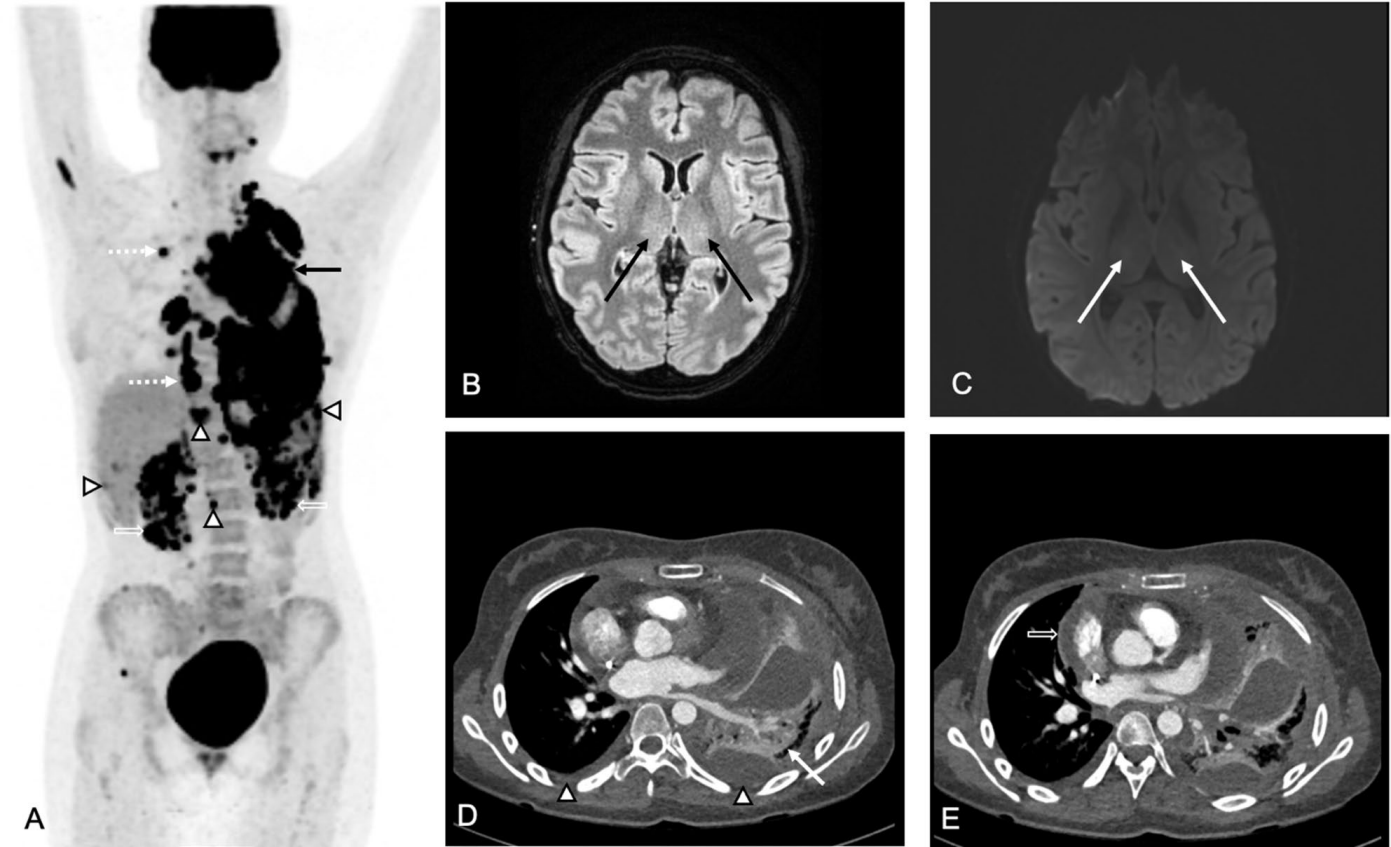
19-year-old female with diffuse large B-cell lymphoma and high baseline metabolic tumor burden who developed grade 2 cytokine release syndrome (CRS) and grade 3 immune effector cell–associated neurotoxicity syndrome (ICANS) after CAR T-cell therapy. A) 18F-FDG PET MIP at baseline demonstrates a bulky hypermetabolic anterior mediastinal mass (black arrow), extensive 18F-FDG-avid lymphadenopathy above and below the diaphragm (dashed arrow arrowheads) as well as multiple hypermetabolic lesions in the bilateral kidneys (open arrows), spleen (open arrowhead), liver (open arrowhead) and bone marrow (open arrowheads). B) Axial FLAIR MR image of the brain at day 6 after the CAR T-cell injection shows mild bilateral thalamic T2-hyperintensity (black arrows). C) Diffusion-weighted MR image of the brain at day 6 reveals subtle restricted diffusion in the bilateral thalami (white arrows). D) Axial contrast-enhanced CT image demonstrates a small right pleural effusion and a moderate left pleural effusion (arrow heads), consistent with CRS-related capillary leak syndrome. The adjacent lung demonstrates normal contrast enhancement, consistent with atelectasis rather than pneumonia (white arrow). E) Axial contrast-enhanced CT image through the level of the heart demonstrates associated pericardial effusion (open arrow)
